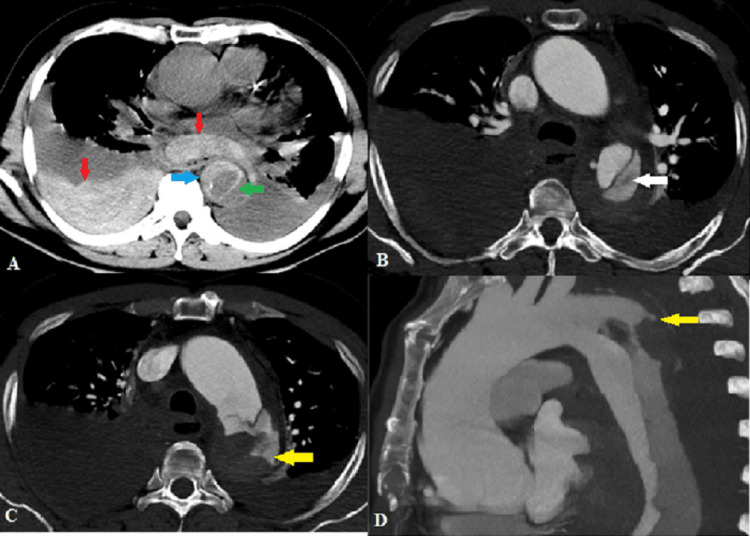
(A) Axial non-contrast CT shows a crescentic IMH (blue arrow) and dissecting intimal flap (green arrow) in the descending thoracic aorta with hemomediastinum and right hemothorax (red arrows). Axial CT angiography images show (B) dissecting intimal flap with faintly opacifying false lumen (white arrow) and (C) probable site of rupture along the left lateral wall of the aorta (yellow arrow). (D) Sagittal MIP image shows the site of rupture. IMH: intramural hematoma; MIP: maximum intensity projection.
